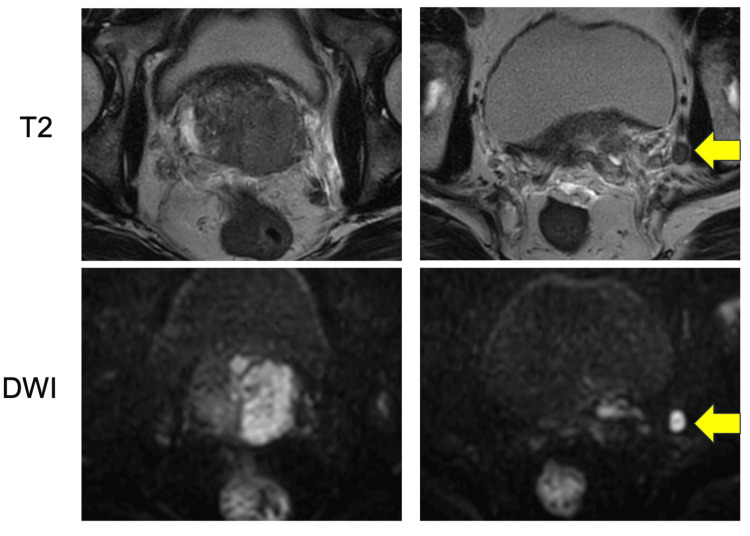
MRI revealing prostate cancer and left pelvic lymph node involvement before treatment initiation. The arrows show enlarged left pelvic lymph node. DWI: diffusion-weighted imaging
